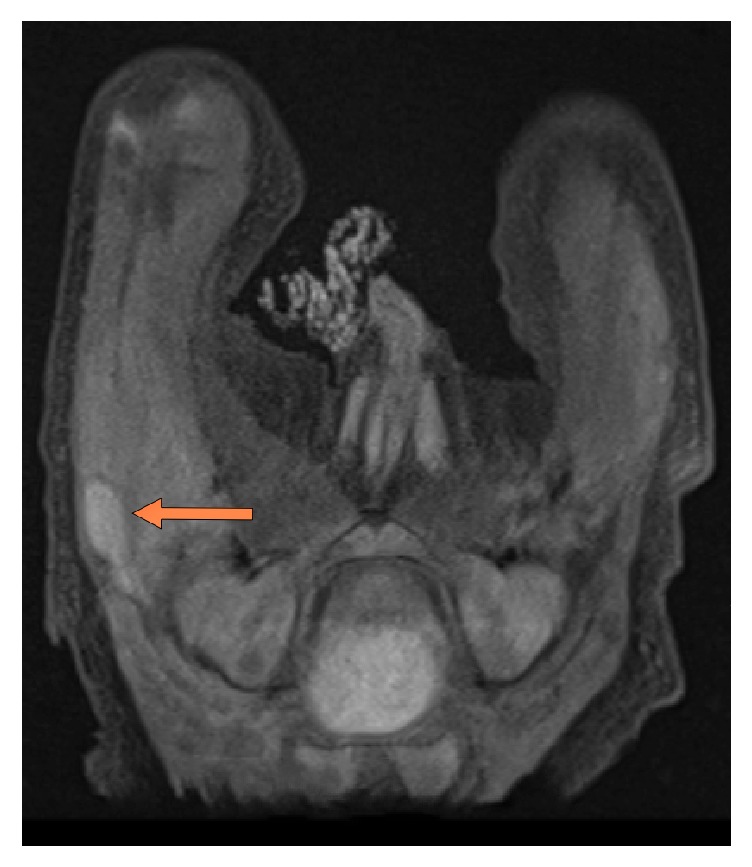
MRI image axial cut demonstrating deep subcutaneous fluid collection with peripheral rim enhancement (arrow) concerning for soft tissue abscess.
